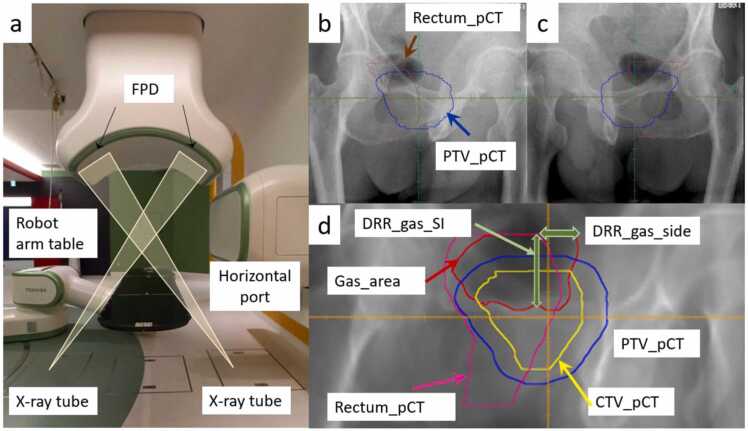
Images to complement the method. (a) Arrangement of an irradiation room, oblique kV-x-ray image system; (b), (c) kV-x-ray images (35° and 325°, respectively); (d) defined indicator on digital reconstruction radiograph. Abbreviations: DRR, digital reconstruction radiograph; pCT, treatment planning CT; PTV, planning target volume; and SI, superior-inferior.
